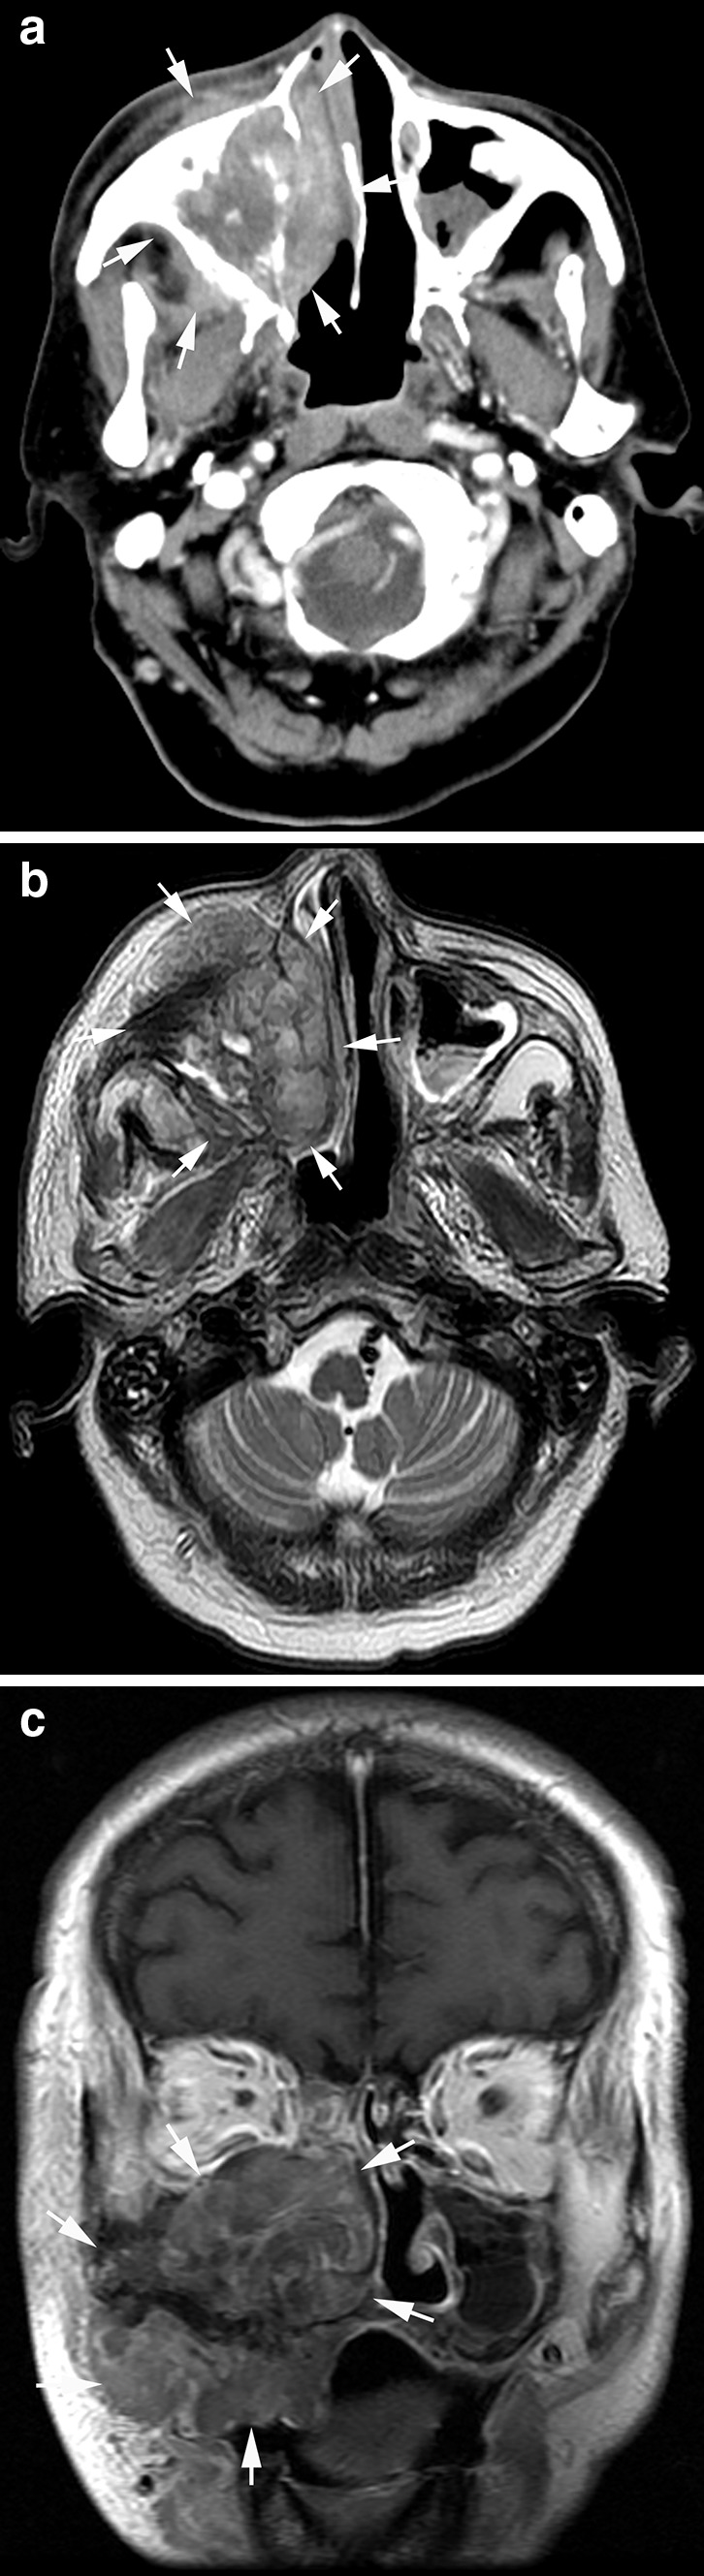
Radiation-induced osteosarcoma 17 years after radiotherapy of a 58-year-old man. a Axial contrast-enhanced CT image showed a large heterogeneous tumor in the right maxillary sinus invading the nasal cavity (*arrows*); b axial T2-weighted image showed heterogeneously high T2 signal intensity of the tumor; c coronal T1-weighted contrast-enhanced image showed the mildly enhanced tumor with wide invasion into the right maxillary region (*arrows*)
